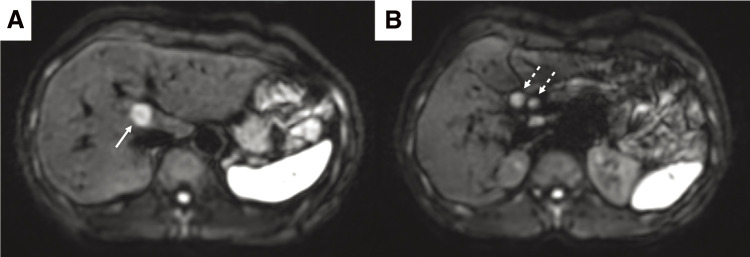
Magnetic resonance imaging findings before chemotherapy. (A, B) On diffusion-weighted images, both the liver tumor (arrow) and enlarged lymph nodes (dotted arrow) show diffusion restriction.
